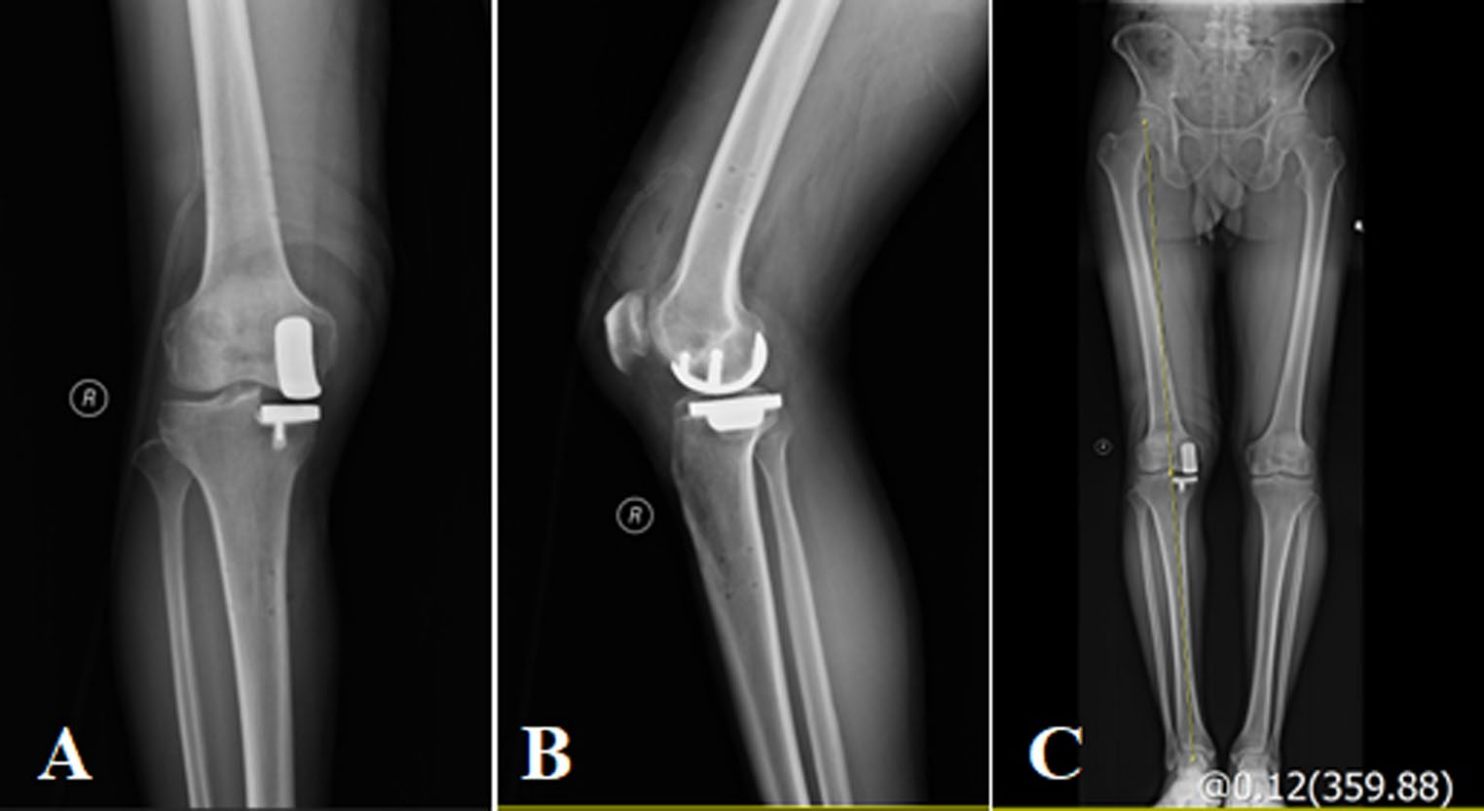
A, B Postoperative anterolateral radiographs of the right knee joint showed that the prosthesis was in good position; C Full-length radiographs of the lower extremity showed good lines of force in the right lower extremity
